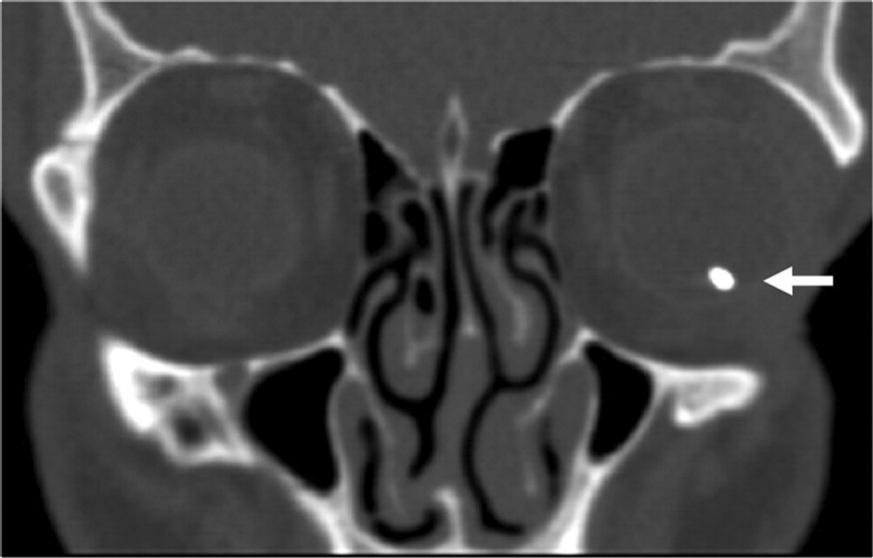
Patient with ferrous IOFB. CT coronal-section image shows a 4-mm metallic IOFB (arrow) inside the left globe. Reproduced with the permission from Pinto et al. (2012). Role of computed tomography in the assessment of intraorbital foreign bodies. Semin Ultrasound CT MR 33 (5):392–395. 10.1053/j.sult.2012.06.004 under CC-BY-NC-ND license
